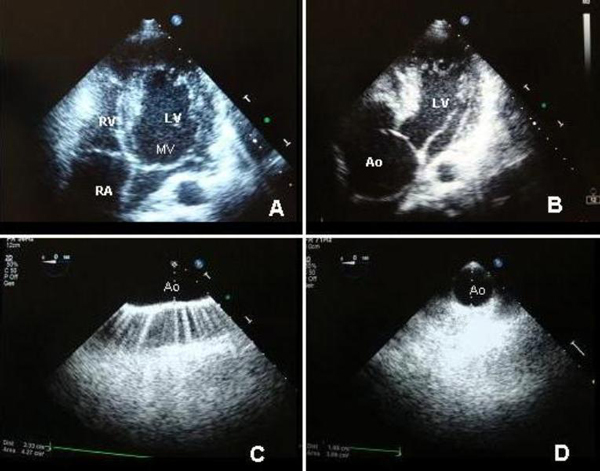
Tansthoracic and transesophageal echocardiography images. (A) Four chamber apical view demonstrating mitral valve prolapse of the anterior leaflet. (B) Apical view of the heart showing the large size of the aorta compared to the left ventricle. (C) Transesophageal echocardiography presents a normal size aortic arch and (D) descending aorta.
